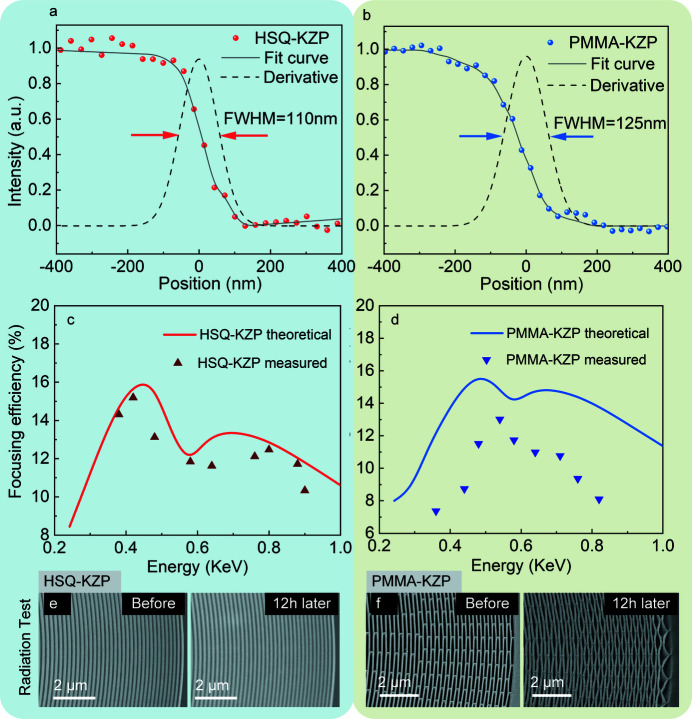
(*a*, *b*) Focusing spot profiles for soft X-rays by the HSQ-KZP and the PMMA-KZP lens measured by knife-edge scan method with dwell time of 1 ms and step size of 25 nm, where the dots are the raw data, the solid lines are the fitting curves and the dash lines are the first-order differentials of the raw data. The first-order derivatives of the scanned curves give rise to a full width at half-maximum (FWHM) of 110 nm for the HSQ-KZP lens and 125 nm for the PMMA-KZP lens. (*c*) Comparison between the measured focusing efficiencies of HSQ-KZP and the theoretical ones using the BPM-QDHT approach. (*d*) Measured focusing efficiencies of the PMMA-KZP lens compared with its theoretical value calculated by the BPM-QDHT approach. (*e*, *f*) Comparison of X-ray radiation damage to the dielectric zone plates by the SEM images of the outermost zones in the HSQ-KZP lens and the PMMA-KZP lens, after 12 h soft X-ray radiation in the tests.
